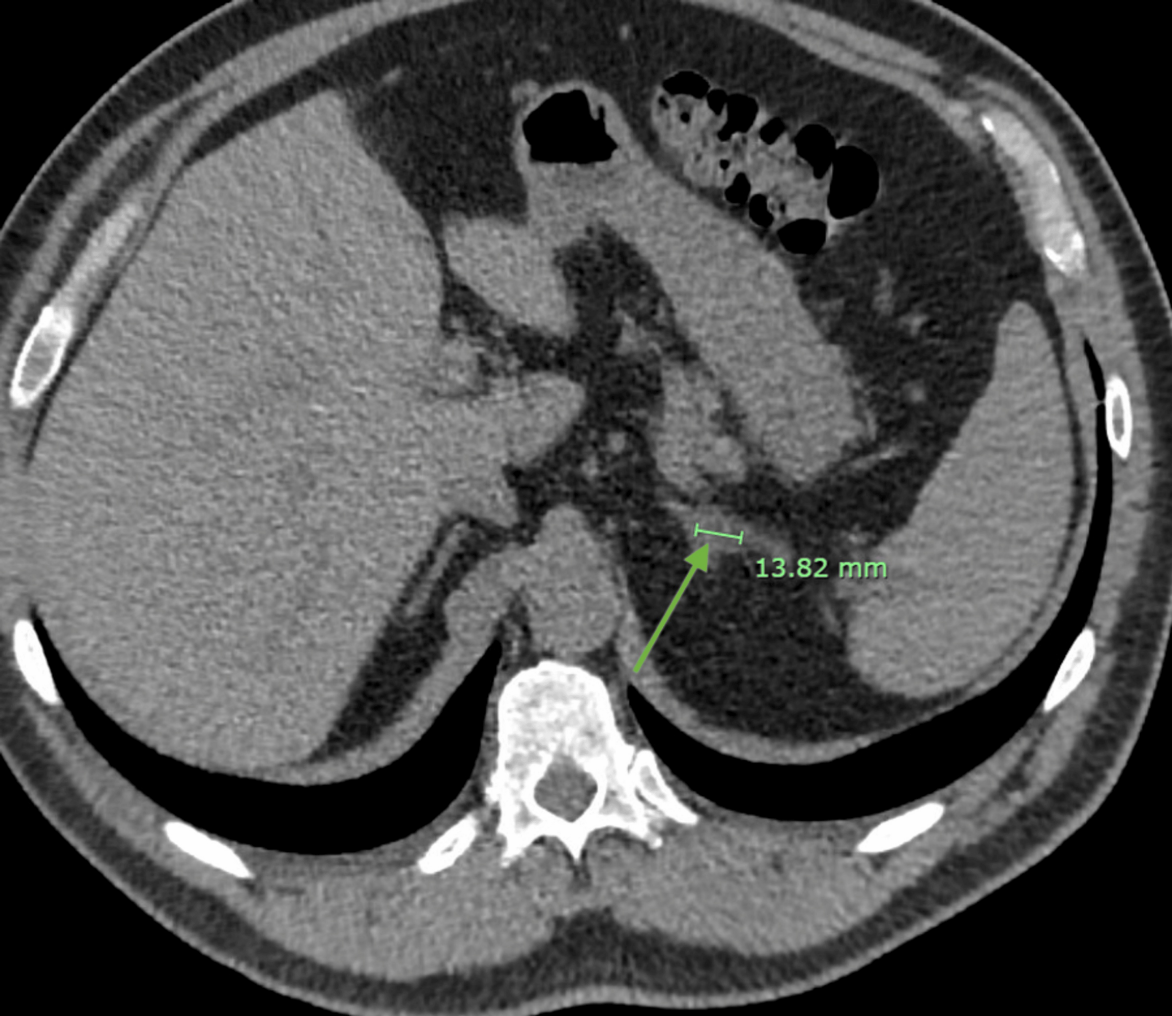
CT abdomen showing adrenal nodule (green arrow).
